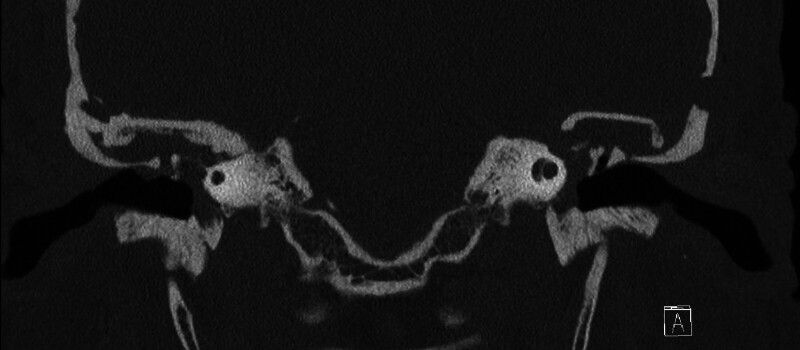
Postoperative computed tomography showing the malleus and tegmen defect covered with a “bony igloo” and AlloDerm.
